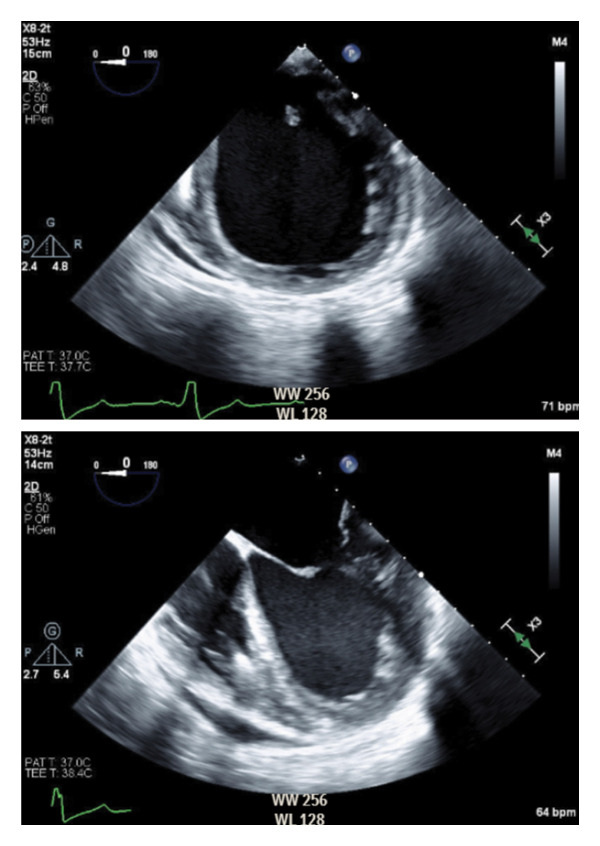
Transesophageal echocardiography images prior to orthotopic heart transplantation displaying a severely dilated left ventricle with severely reduced left ventricular systolic function.
